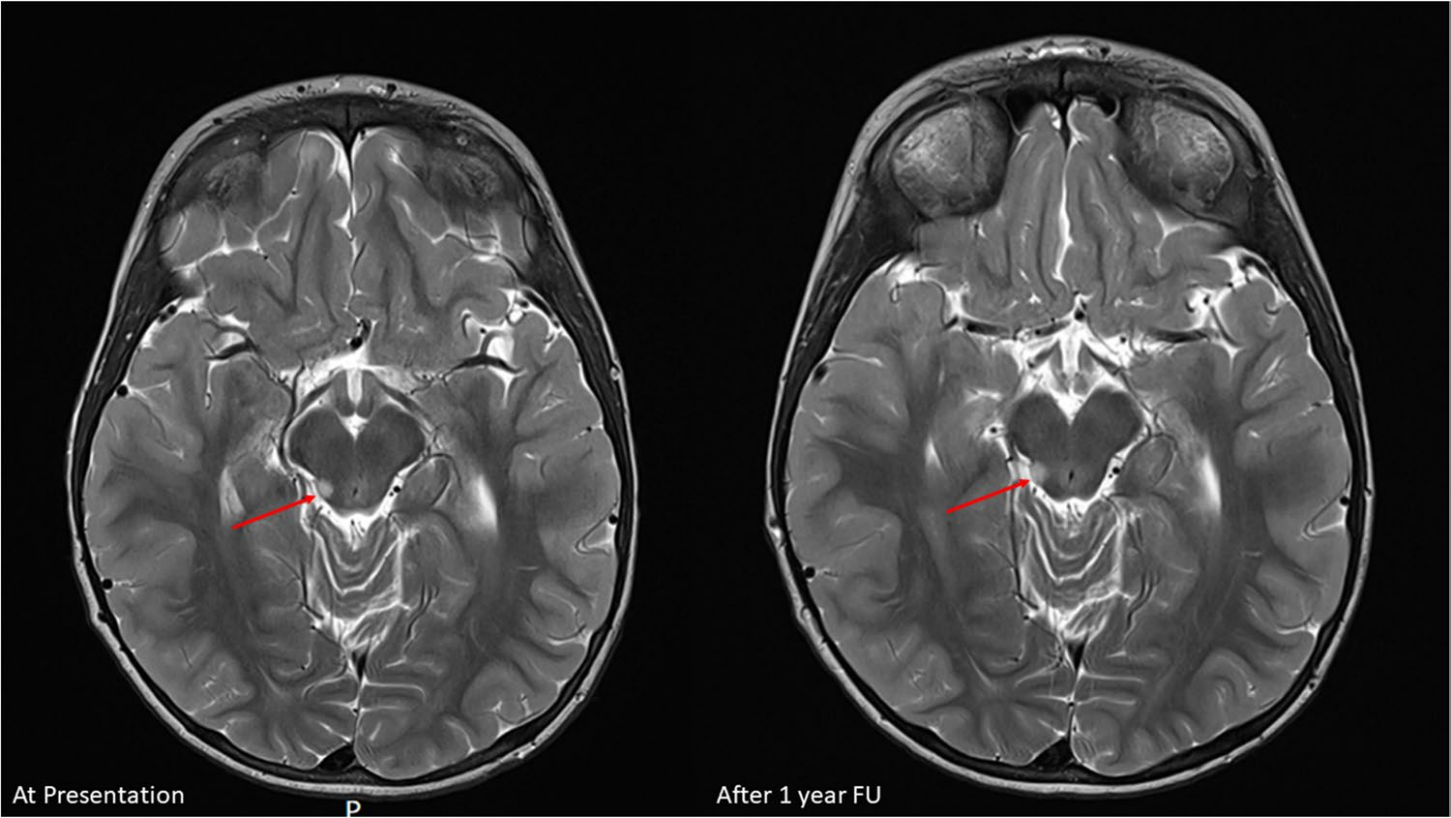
Example of a brain stem incidental brain tumor finding 10-year-old boy presenting with growth retardation. On MRI incidental finding in the right posterior mesencephalon. Conservative management with wait scan follow up was initiated, showing a stable lesion after 1 year. The boy remains neurologically intact
